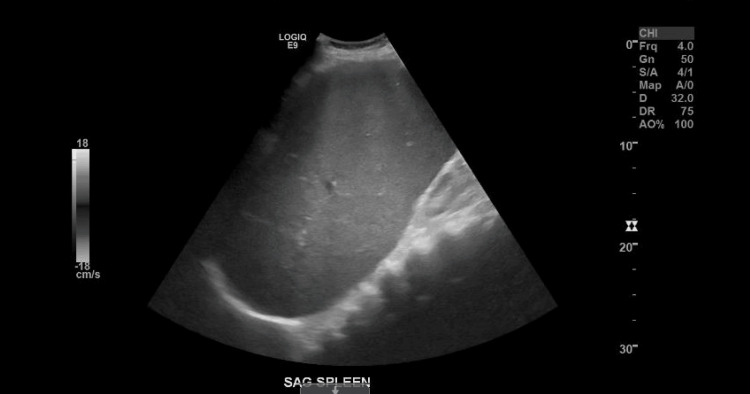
Abdominal ultrasound showing splenomegaly of 29 cm. SAG: sagittal
